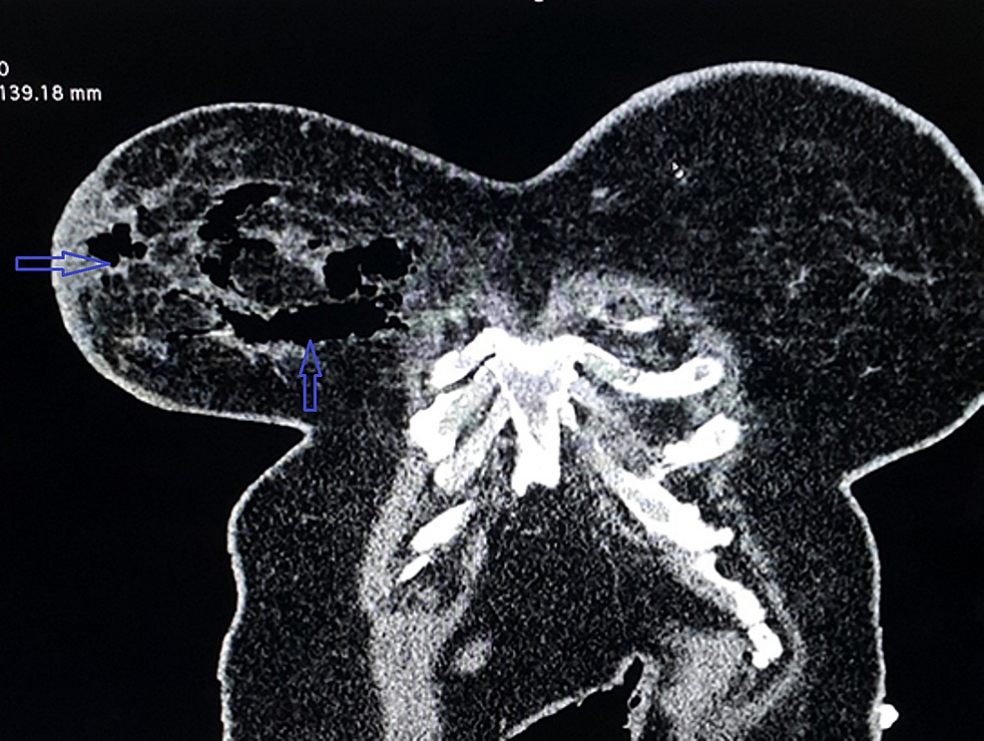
CT pulmonary angiogram showing large pockets of gas within the right breast parenchyma (blue arrows) with edema of the overlying skin CT: computed tomography
